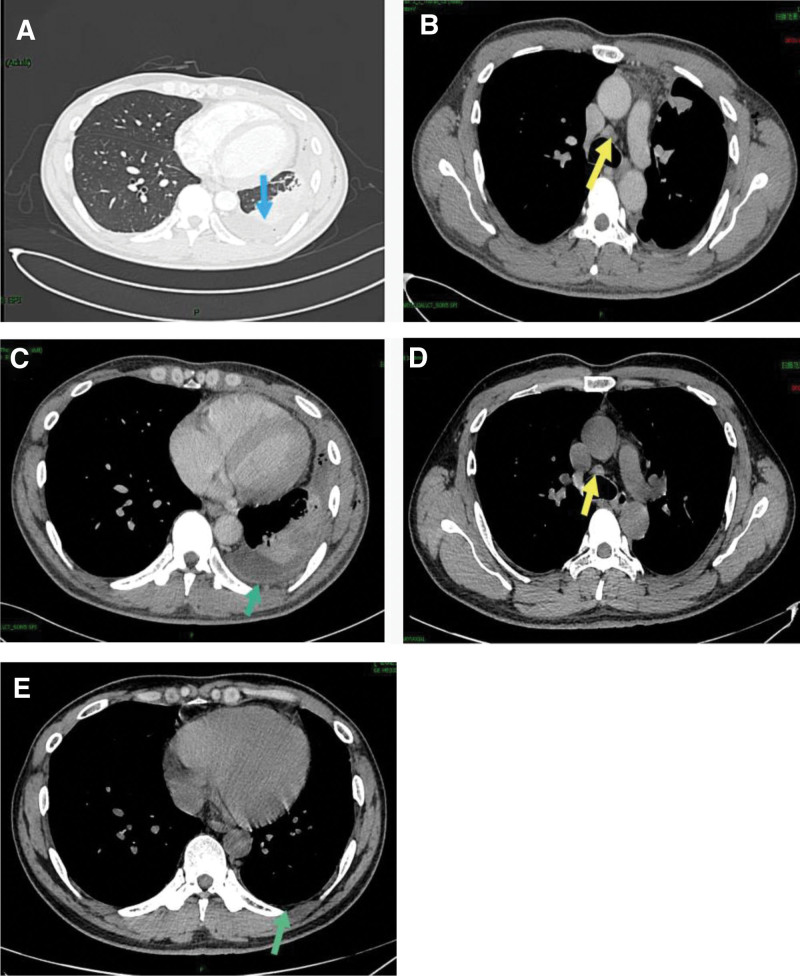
Enhanced chest and abdomen CT: left pleural effusion (blue arrow in A), enlarged lymph nodes in the mediastinum (yellow arrow in B), atelectasis; Pleural thickening at the left (green arrow in C); and changes in mediastinal lymph nodes and pleural at D and E after treatment.
